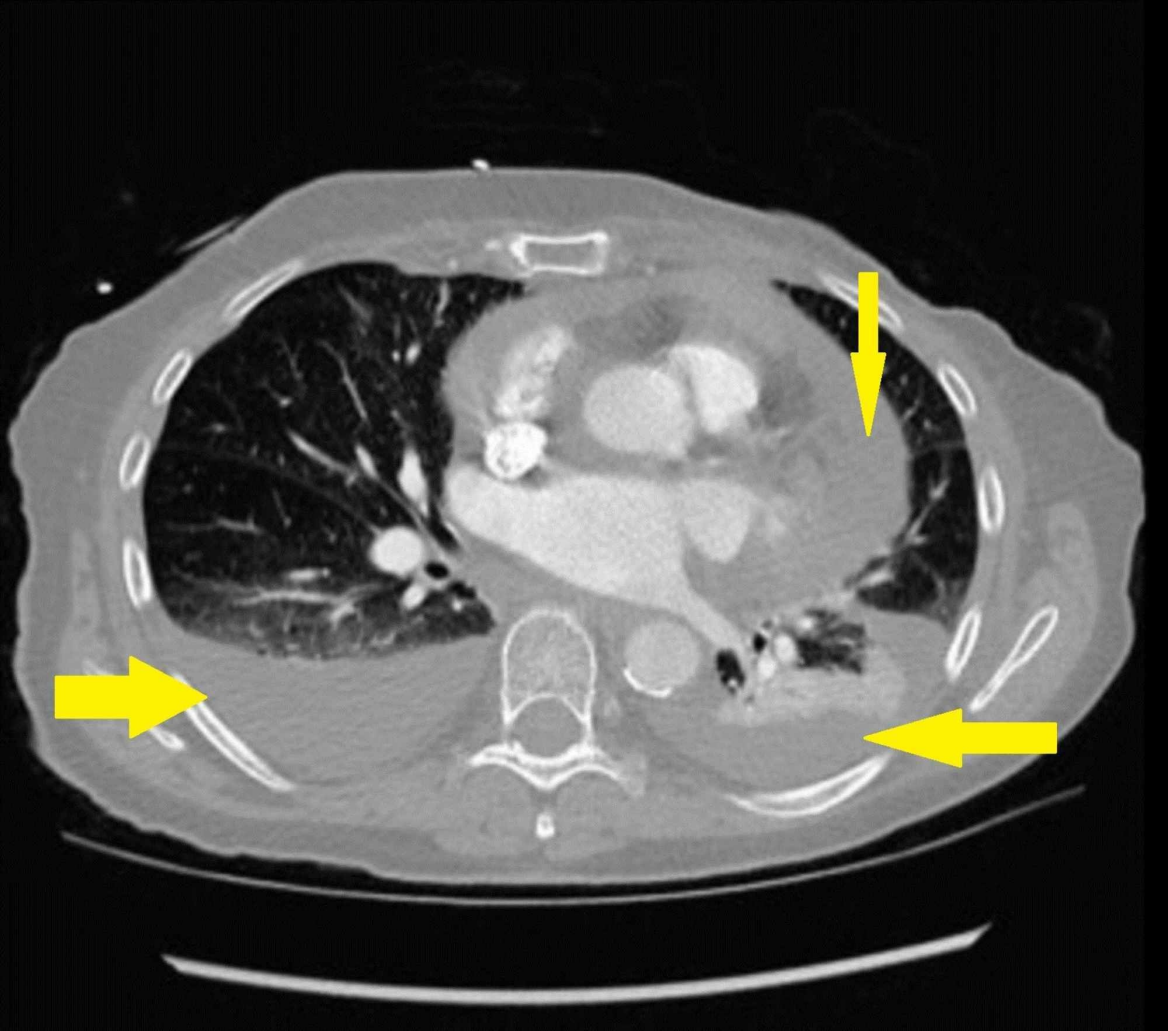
CT Chest Scan - Bilateral Pleural and Pericardial Effusions CT: Computed Tomography
